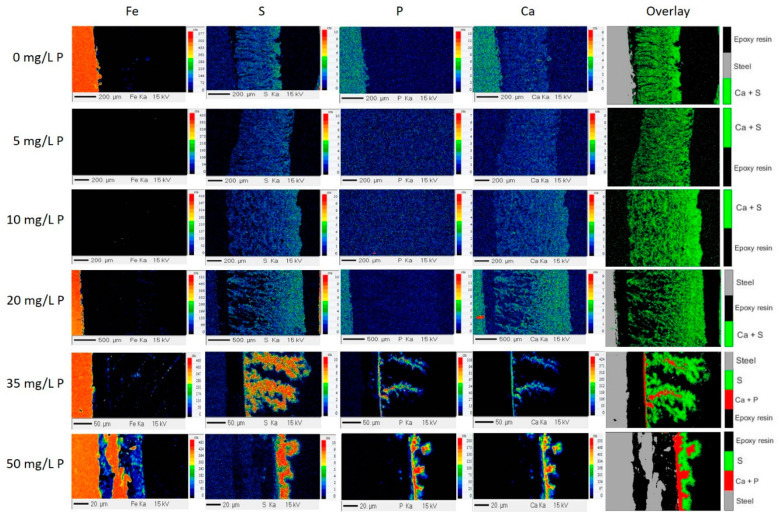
Fe, S, P, Ca, and the overlay X-ray mappings of the cross section of the fouling layer as a function of the added P in the WPI solutions containing 100 mg/L of Ca and 0.5% *w*/*v* of protein. For the samples 5 mg/L P and 10 mg/L P the fouling completely detached from the substrate.
